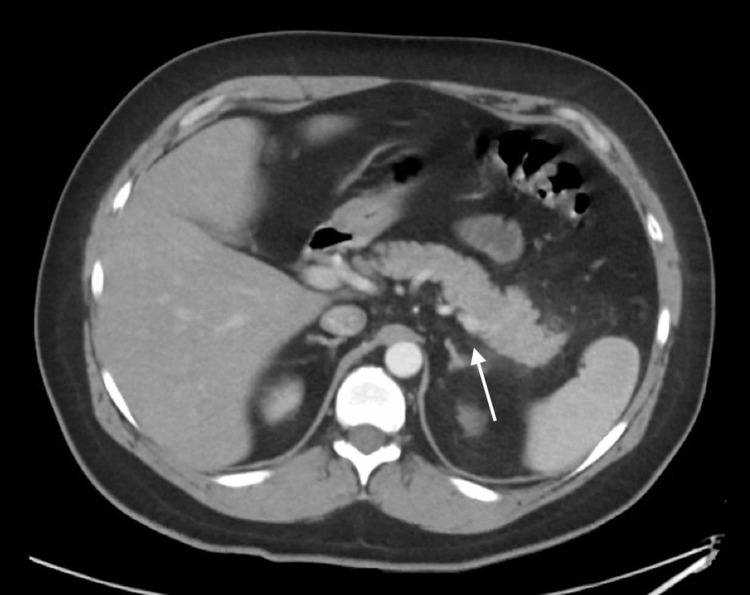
CT imaging demonstrating inflammation of the peripancreatic fat at the body and tail consistent with pancreatitis
